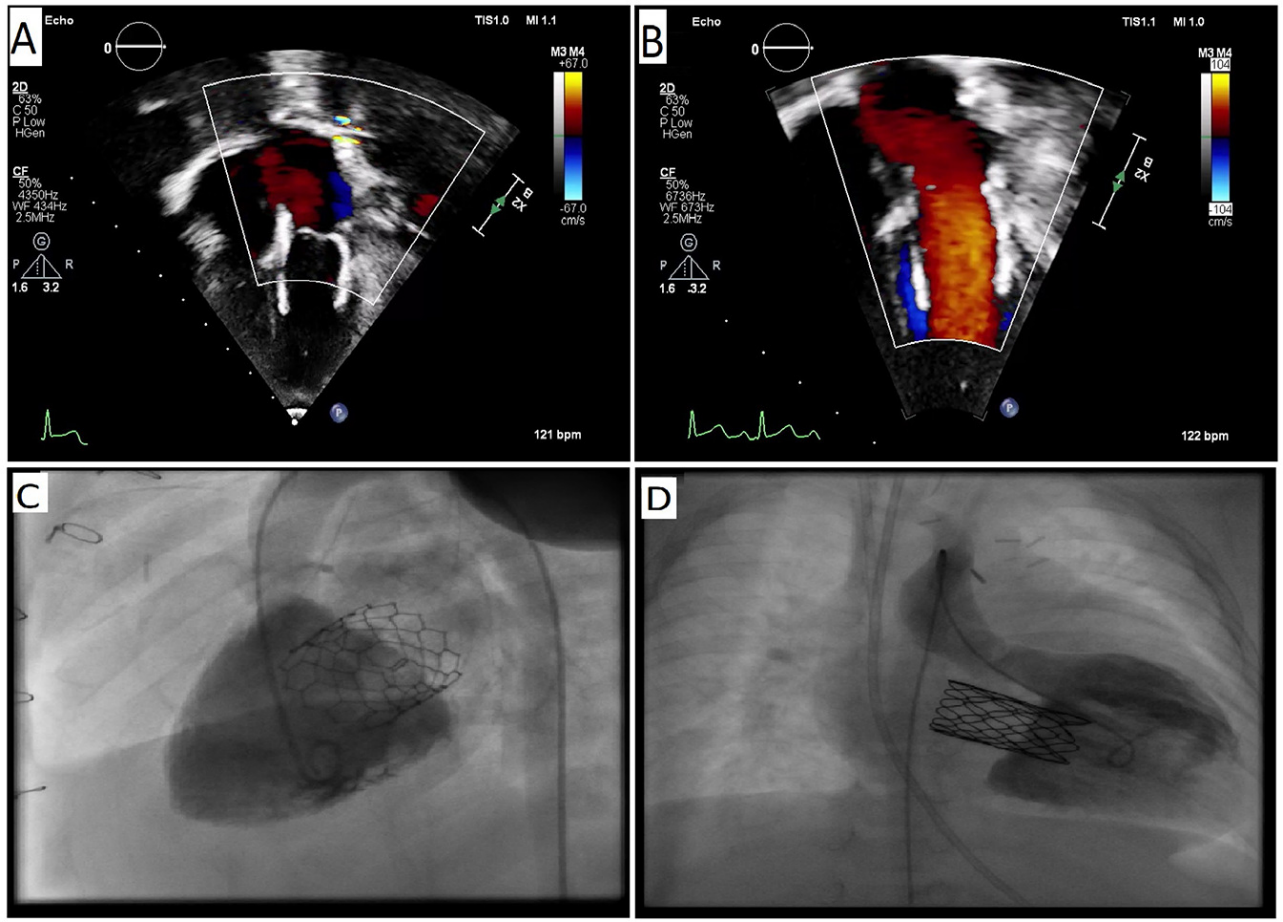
(A) Two-dimensional transthoracic echocardiography, (B) color doppler image of the stent-based valve in mitral position, (C) left ventriculogram showing no mitral regurgitation in Sapien 3 and (D) Melody implanted patients.
